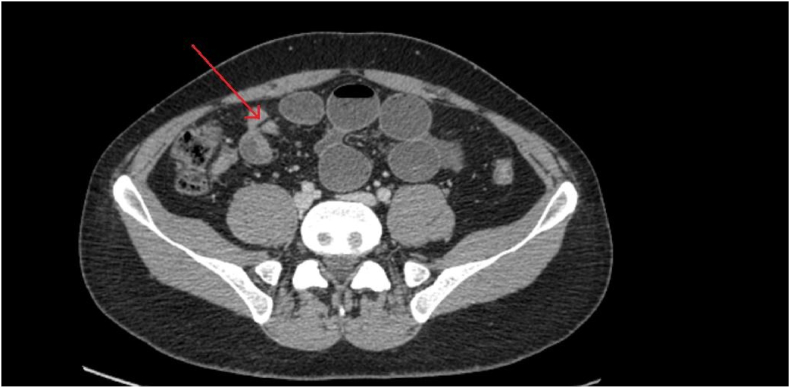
CT scan of the patient's abdomen showing a transition point in the terminal ileum (red arrow), with mesenteric band cut-off. (For interpretation of the references to colour in this figure legend, the reader is referred to the Web version of this article.)
